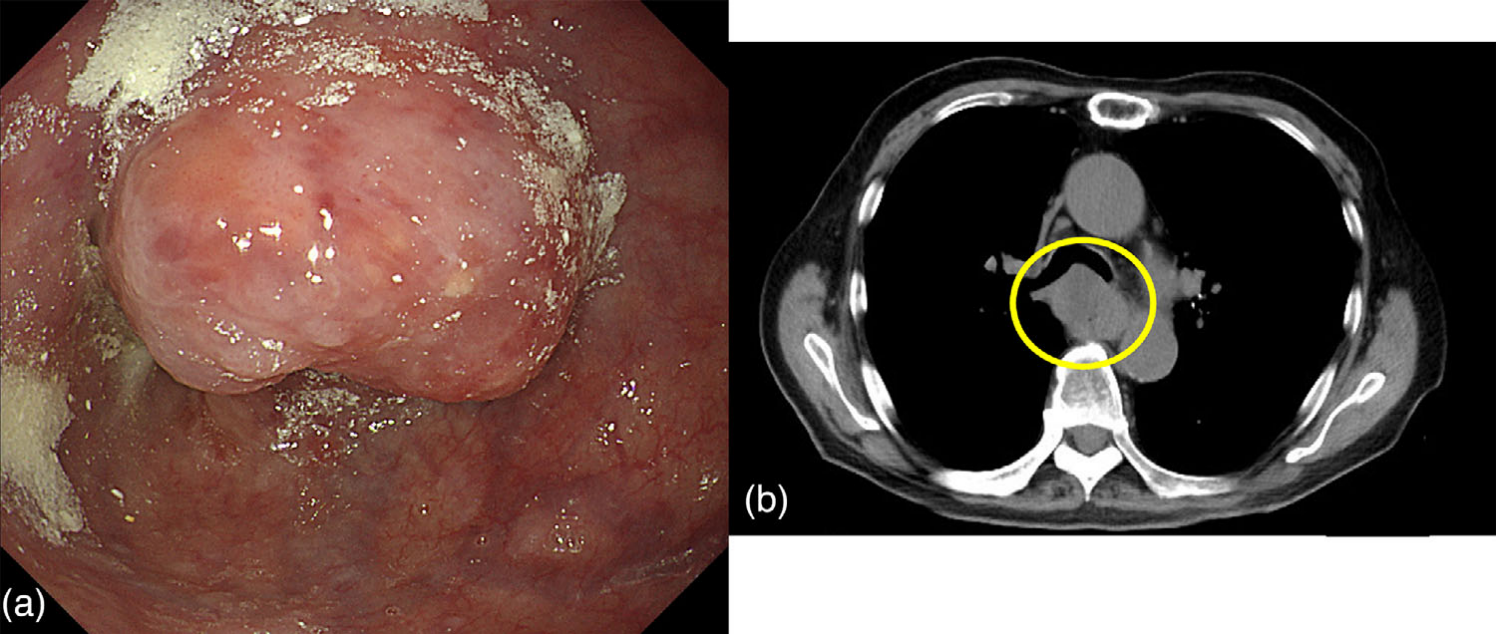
Diagnosis of esophageal squamous cell carcinoma. (a) Upper gastrointestinal endoscopy shows an elevated lesion with the upper wall of the mid‐thoracic esophagus as its main locus. (b) Computed tomography shows the tumor in contact with the bronchus (yellow circle).
